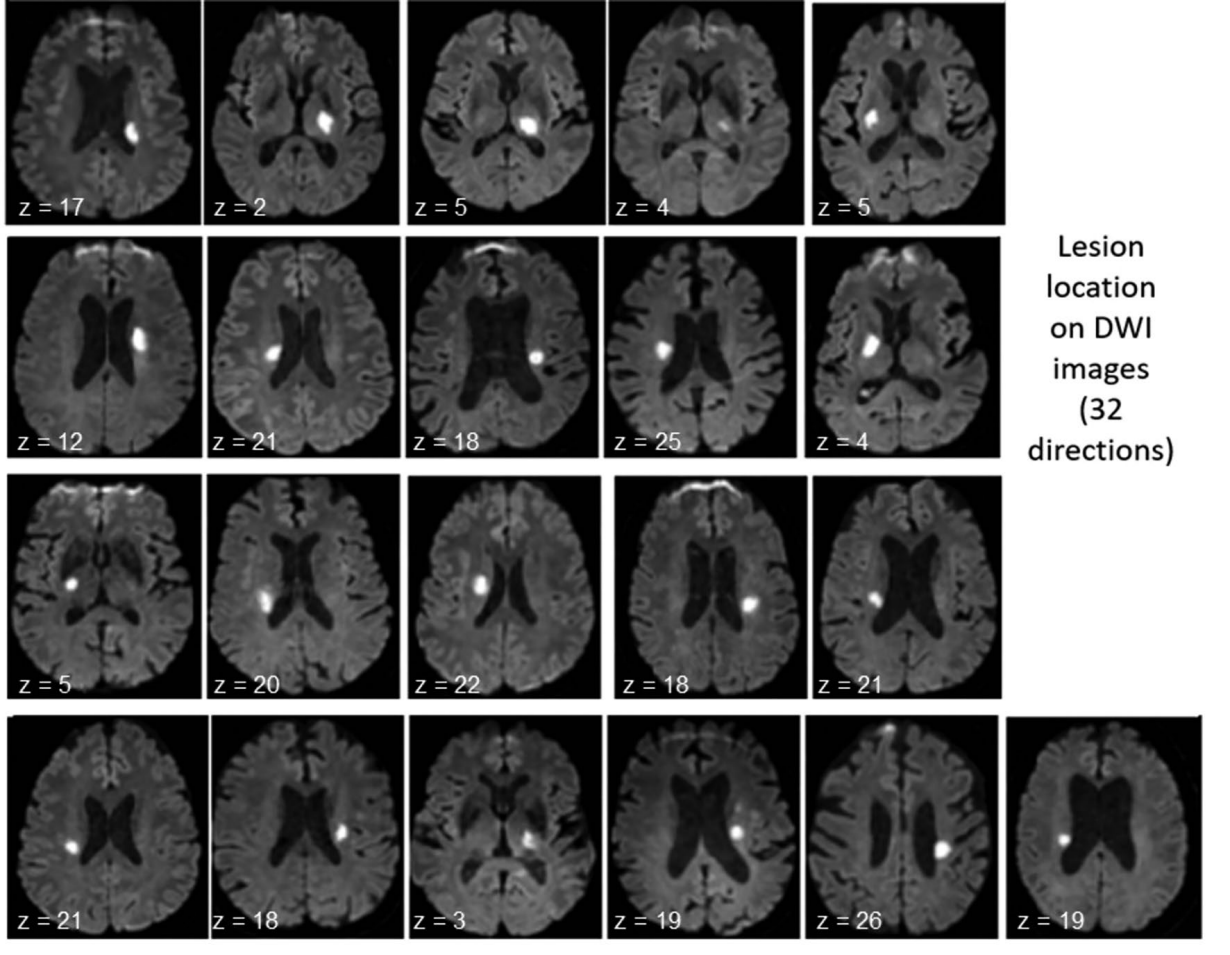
Lesion location on DWI images at baseline (*n* = 21 patients). z in mm above AC–PC plane
